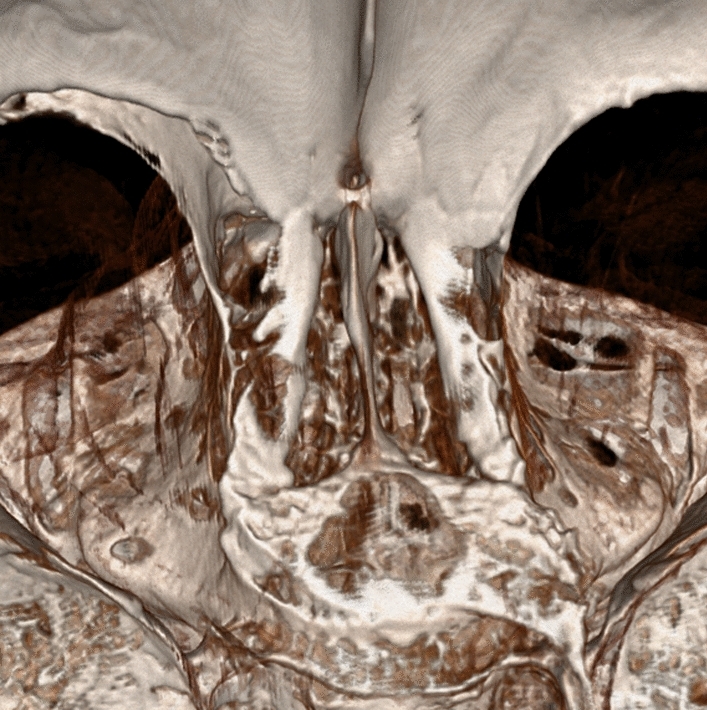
3D Reconstructed CT scan of Cribriform Plate and Crista Galli using SECTRA (Linköping, Sweden) IDS7 Software
